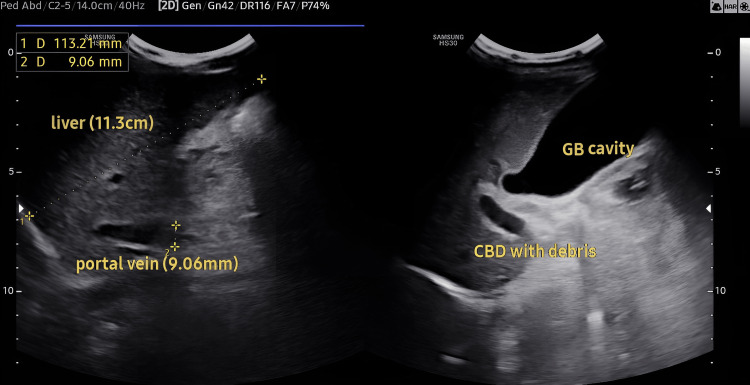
Ultrasonography showing liver enlargement, portal vein, and gallbladder sludge. GB, gallbladder; CBD, common bile duct
